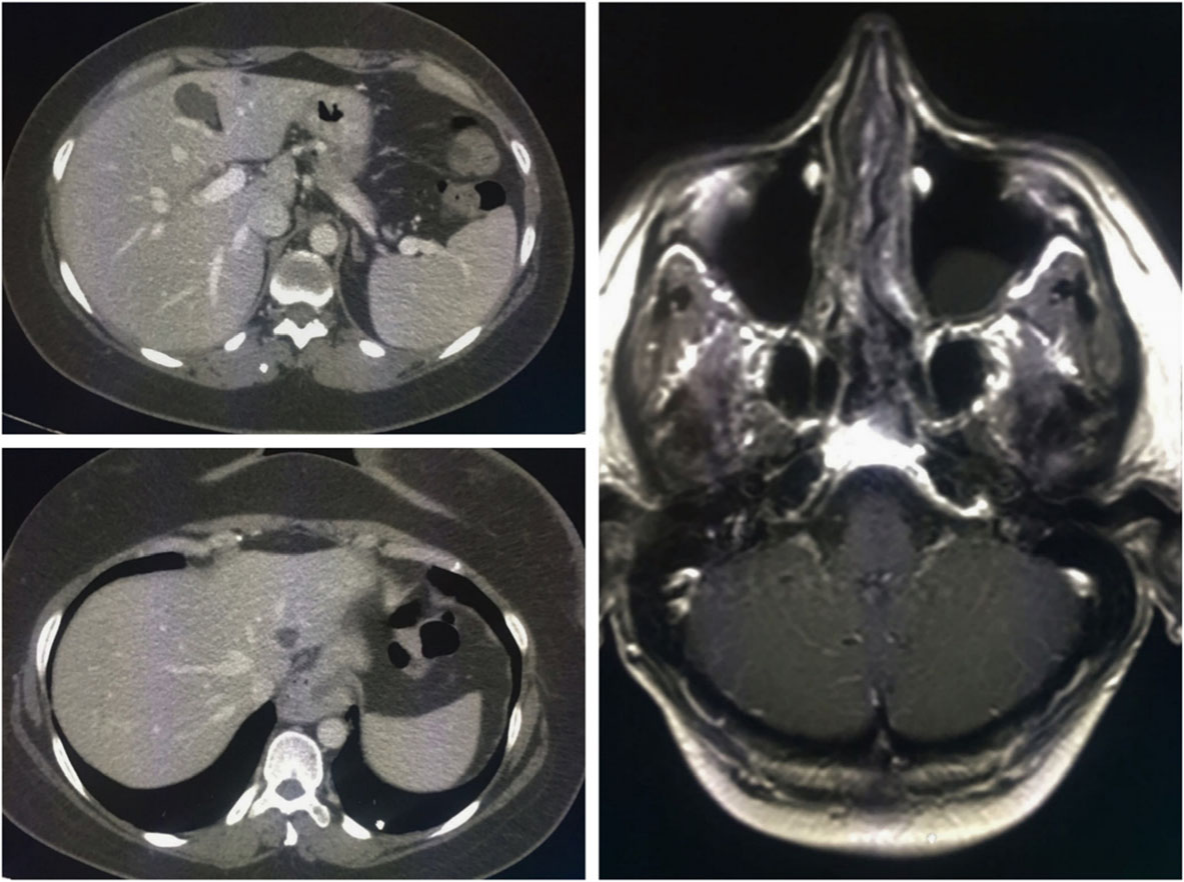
November 2016, the brain MRI confirms the CR in the CNS
